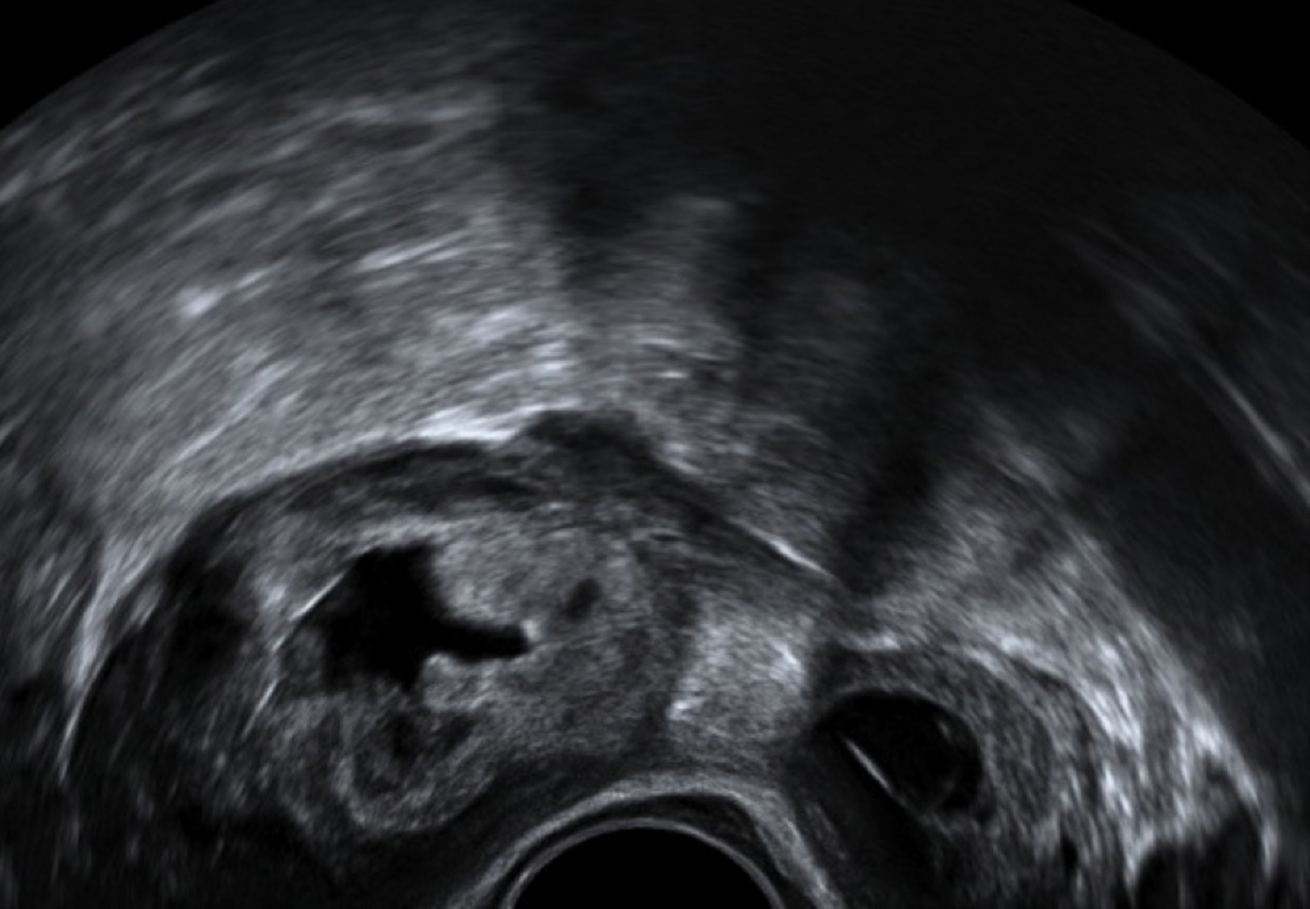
Ultrasound image of a left tubal ectopic at 6+1 weeks gestation. A hyperechoic mass is seen adjacent to the left ovary.
